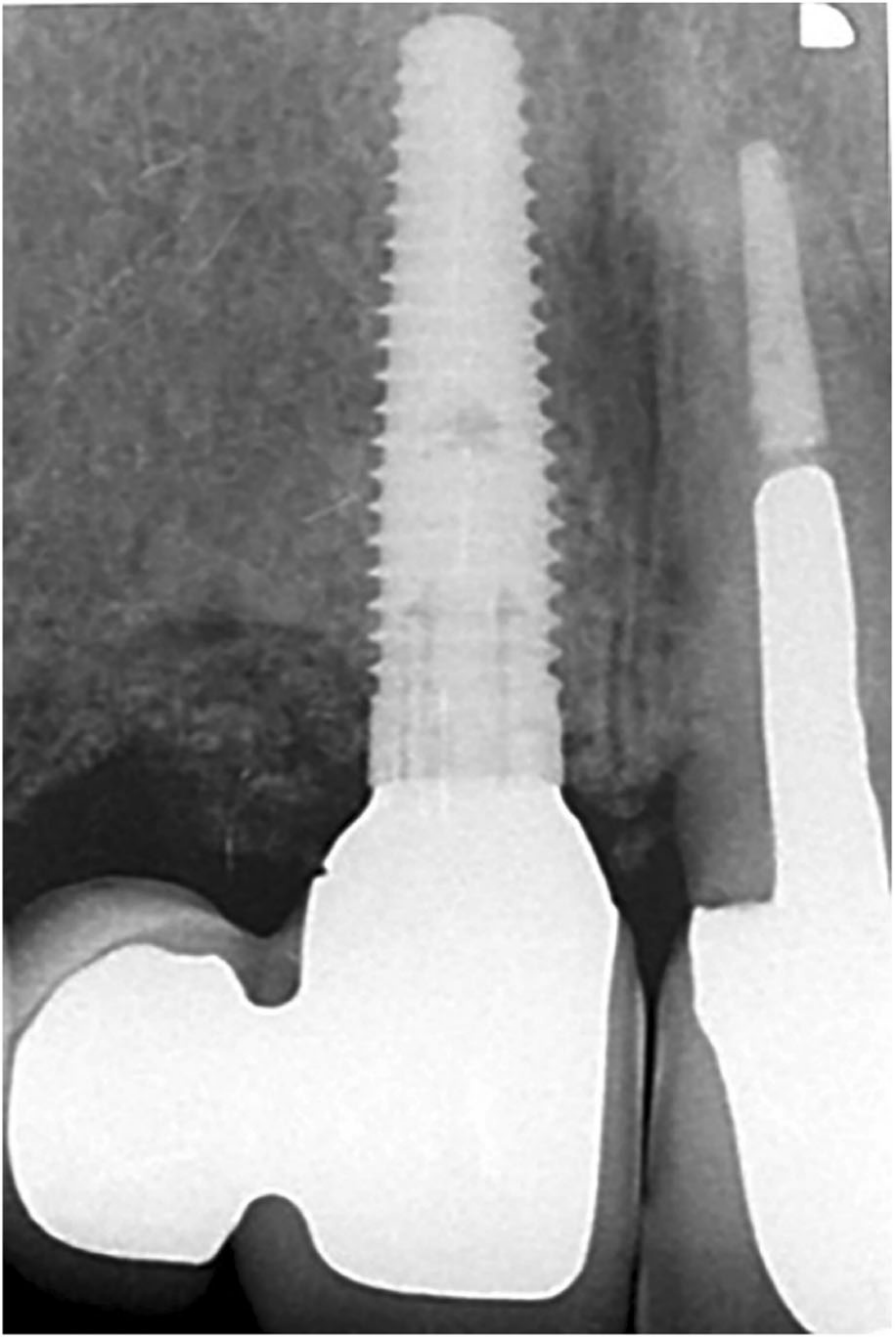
Ten‐years radiograph of implant‐supported two‐unit cantilever crown.
